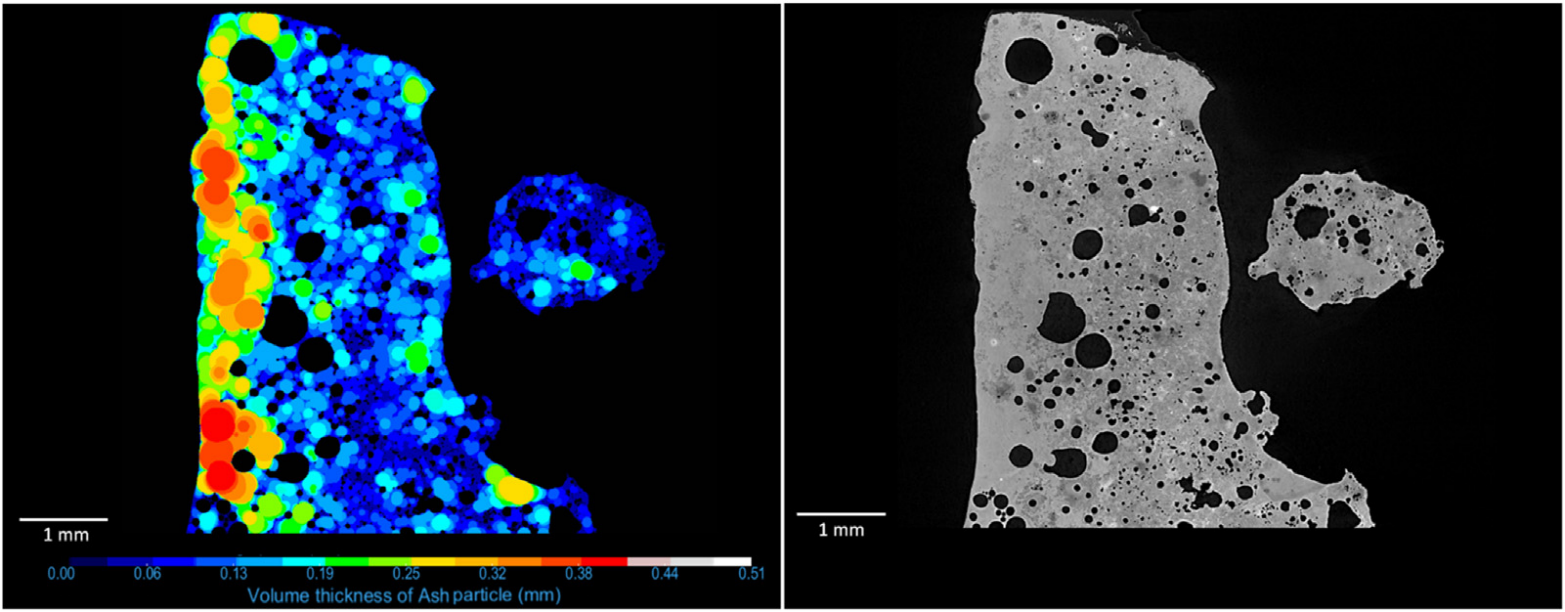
An ortho slice showing volume thickness map of the ash particle (left), coloured after intensity level that indicates the local thickness. To the right the corresponding ortho slice with the grey scale apply to X-ray attenuation intensities.
